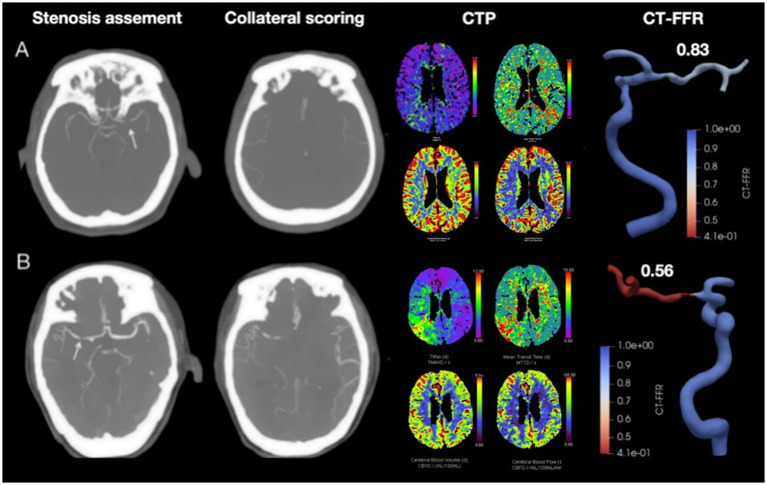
Examples of two patients with severe intracranial artery stenosis. Case 1 (A): classified into the non-recurrent ischemia cohort. CT angiography (CTA) shows 75% stenosis at M1 segment of the left middle cerebral artery (white arrow) and normal prominence of pial arterial compared to contralateral hemispheres (collateral score = 5); CTP demonstrates normal cerebral perfusion, and the value of CT-FFR was 0.83. Case 2 (B): classified into the recurrent ischemia cohort. Atherosclerotic plaque at M1 segment of the right middle cerebral artery results in 87% stenosis (white arrow). Multiphase CTA shows some ischemic regions without visualization of brain vessels (collateral score = 2). Cerebral hypoperfusion in the right temporo-parietal lobe on CTP (increase in Tmax and MTT, reduction in CBF, and normal CBV) and low CT-FFR (CT-FFR = 0.56) were shown. Tmax, time-to-maximum of the tissue residue function; MTT, mean transit time; CBF, cerebral blood flow; CBV, cerebral blood volume; CT-FFR, CT-derived fractional flow reserve.
